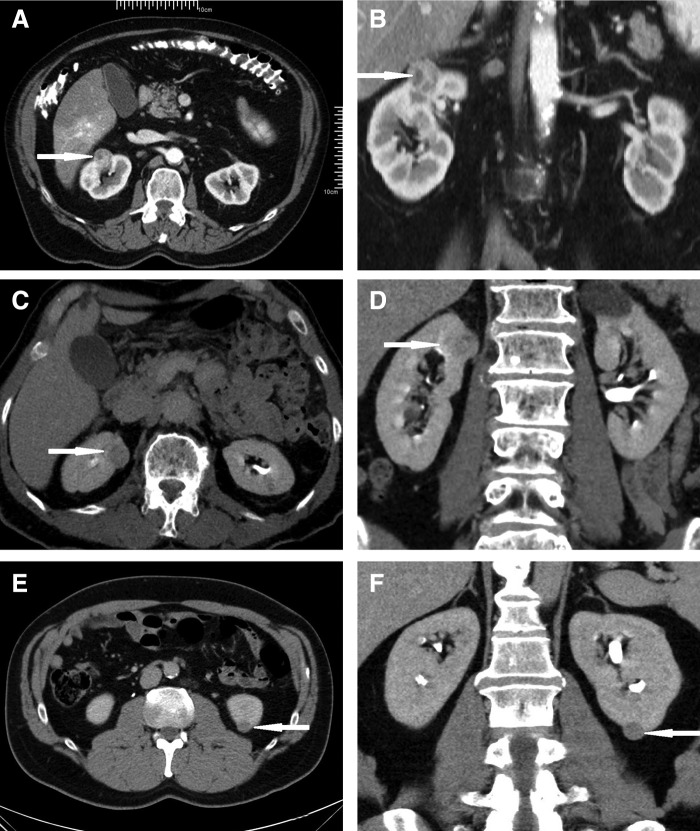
CT images of the patients, with tumors indicated by *arrows*. (A, B) Transverse and coronal views of renal tumor in the first patient. (C, D) Transverse and coronal views of renal tumor in the second patient. (E, F) Transverse and coronal views of renal tumor in the third patient.
